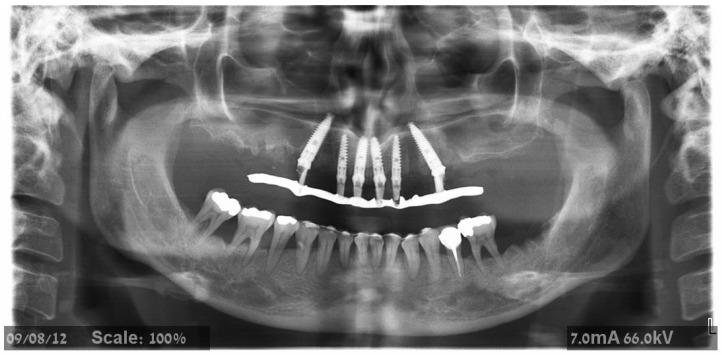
Panoramic X-ray on the day of provisional prosthesis delivery. Two posterior tilted implants were placed on each side to avoid sinus augmentation.
